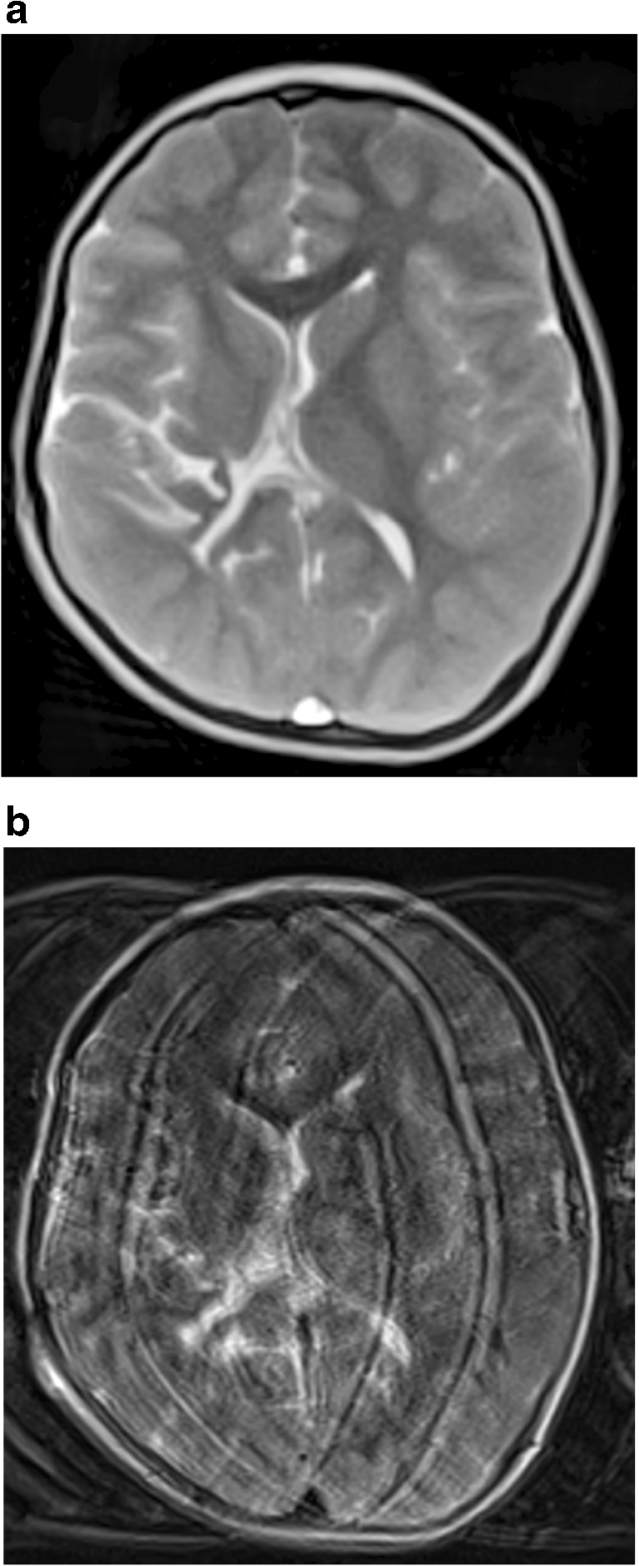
Comparison of axial image quality in the presence of motion in a boy age 4 years 6 months with shunted post-hemorrhagic hydrocephalus. a Volume coverage scan with refocused fast low-angle shot contrast (T2/T1). b Conventional T2-weighted fast spin echo
